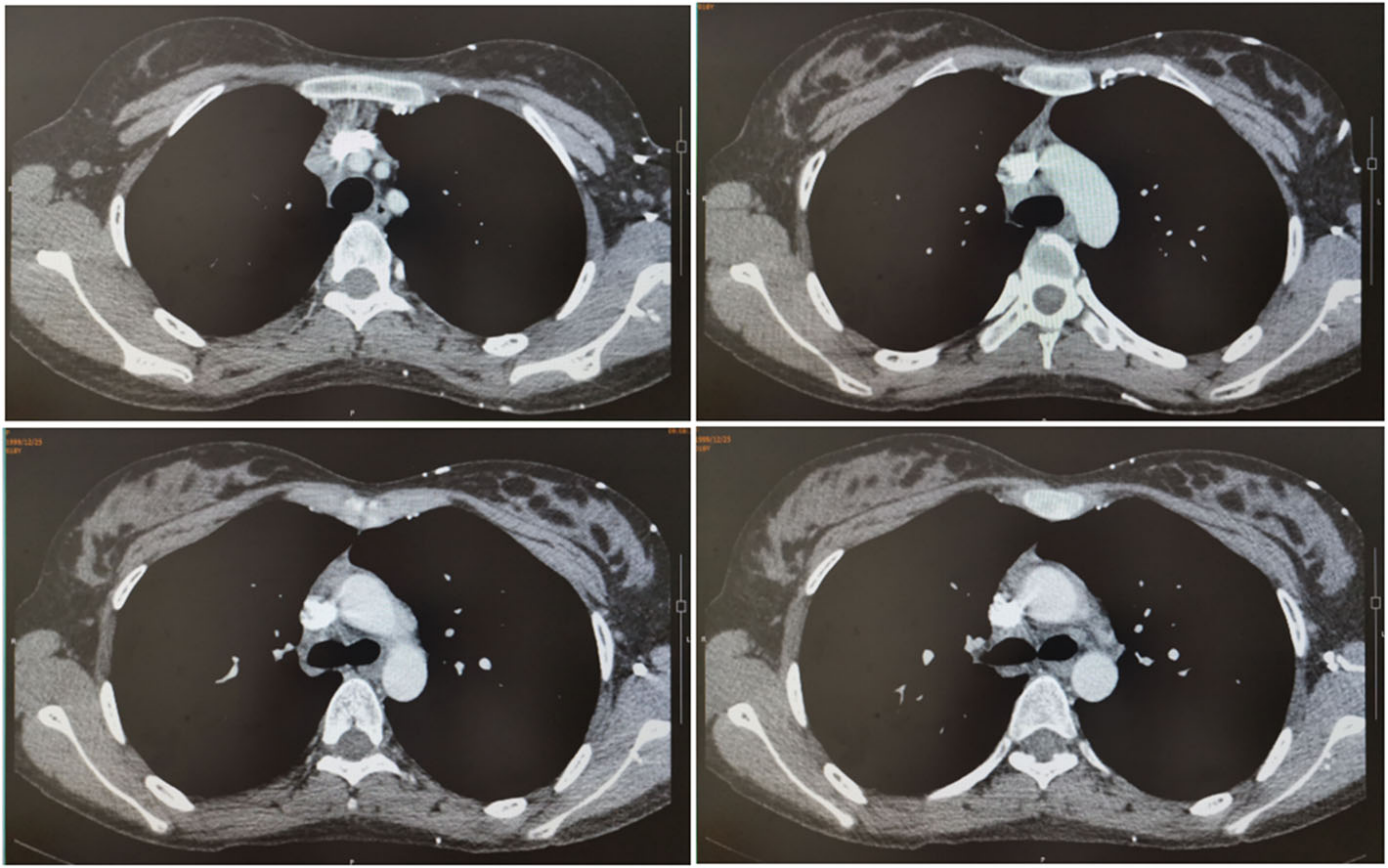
Chest CT reexamination on September 28, 2018
